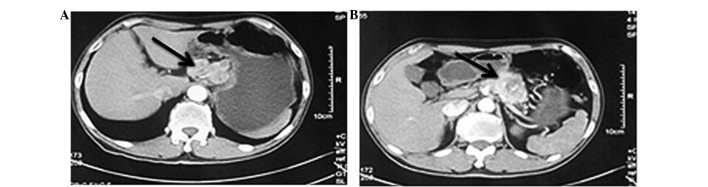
Computed tomography images from patient no. 12, a 60-year-old with a two-month history of abdominal pain, showing masses located on the pancreatic body and tail, with lower density and irregular shadows. (A) The venous phase showing the main pancreatic duct expansion, and (B) the delayed phase with slightly uneven density, showing a patchy low-density area of necrosis; (arrows indicate the location of the tumor).
